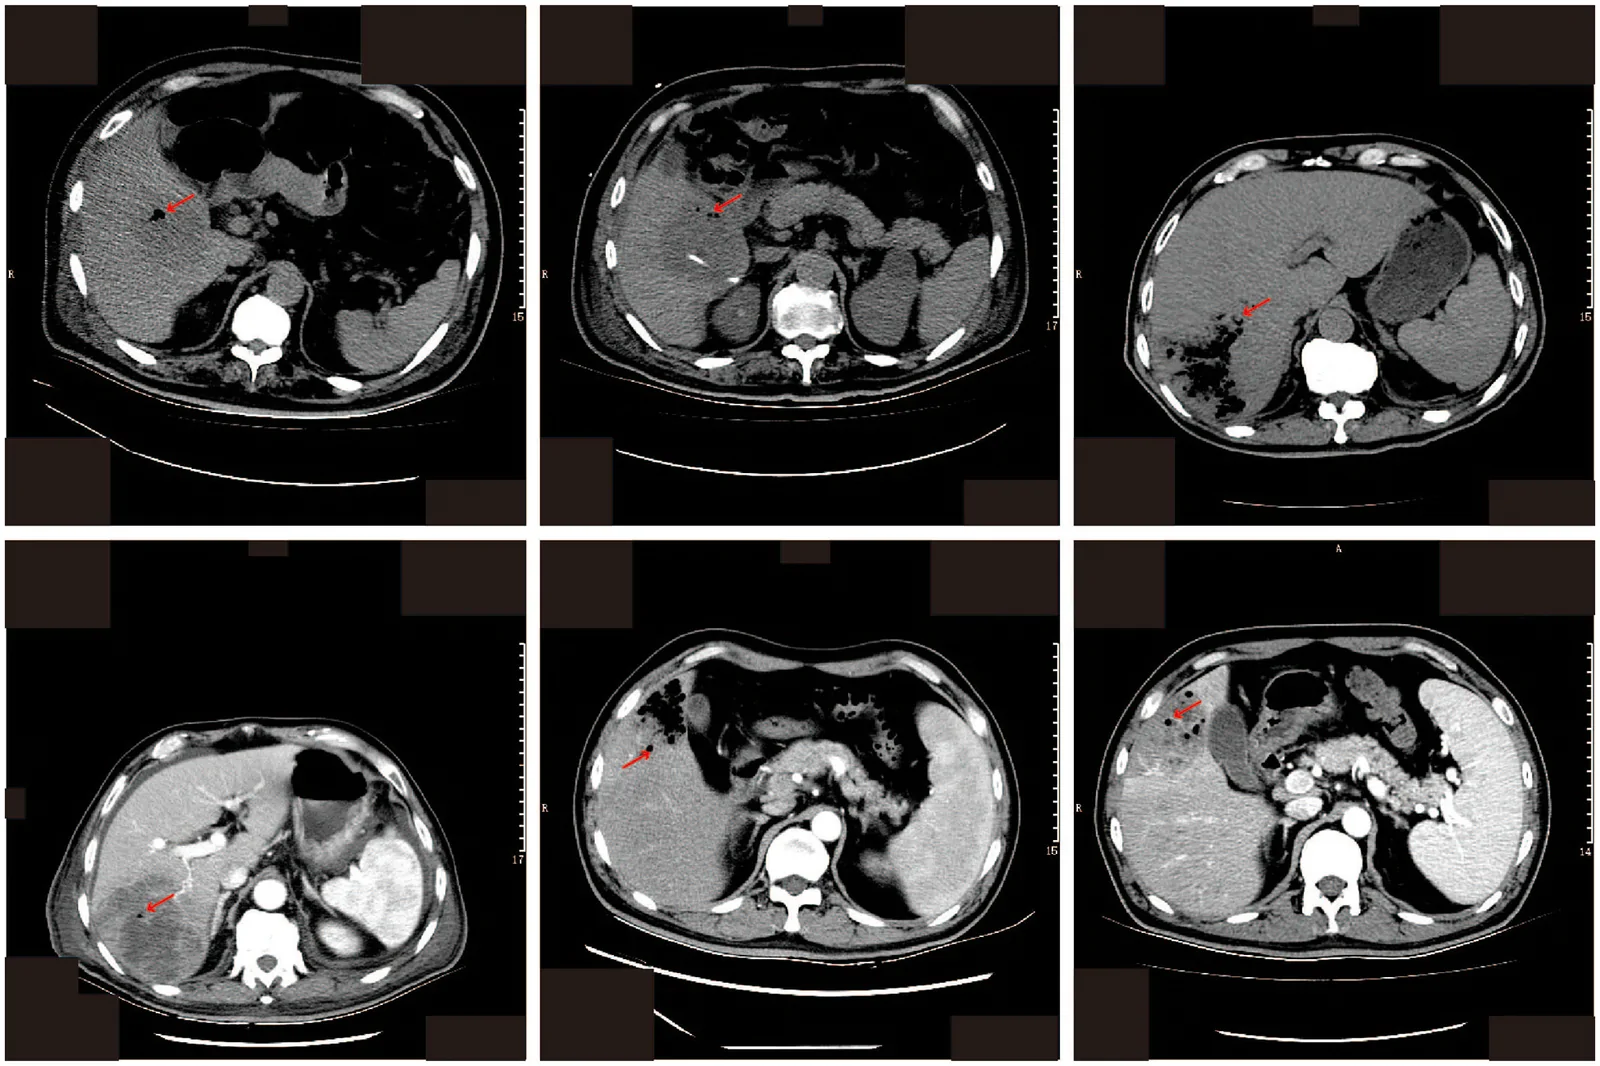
CT manifestations of patients with gas-producing liver abscesses. The red arrow points to the gas.
